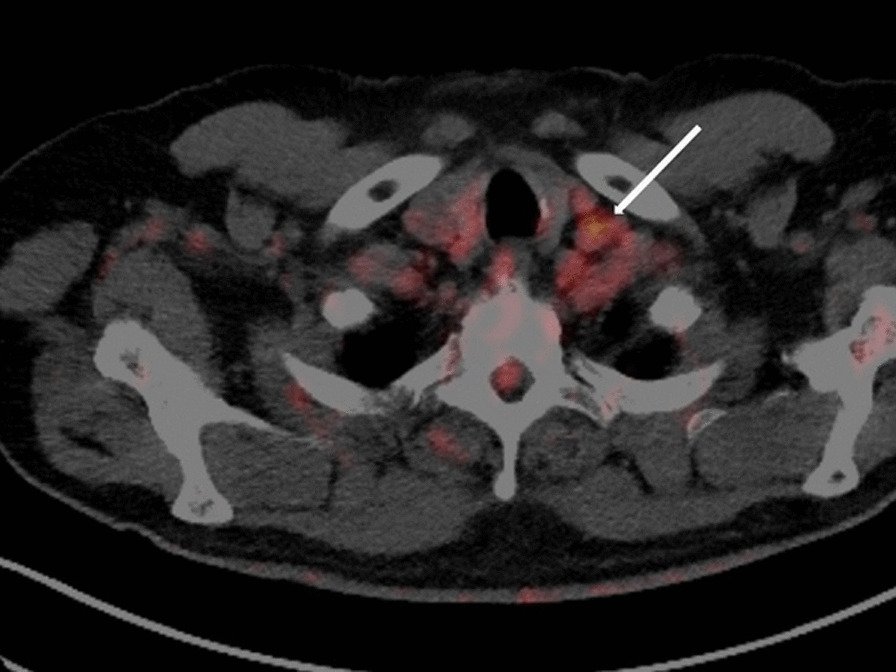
FDG-PET scan showing left supraclavicular lymph nodes with moderate metabolic activity [standardized uptake value (SUV) 4.0]
